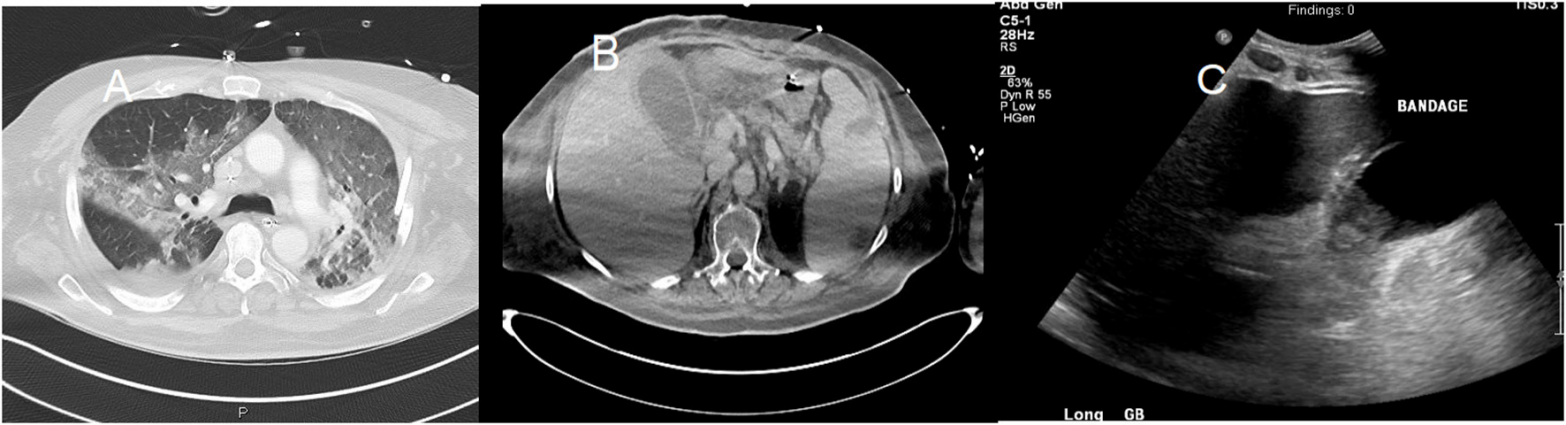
A 58-year-old man with a past medical history of obesity, post-primary coronary intervention, and coronary artery disease, got admitted to the intensive care unit with severe hypoxia and tachycardia. The patient tested positive for COVID-19. (A) Chest CT showed patchy airspace opacities with predominant ground-glass opacities seen in the upper lobes and consolidative opacities with air bronchograms in the lower lobes. (B) Abdominal CT showed hepatosplenomegaly with splenic infarcts and perisplenic fluid collections. Gallbladder wall thickening with trace pericholecystic fluid was also noted, suggesting acalculous cholecystitis. (C) An abdominal ultrasound image of the same patient showed moderately distended and thickened walls of the gall bladder.
